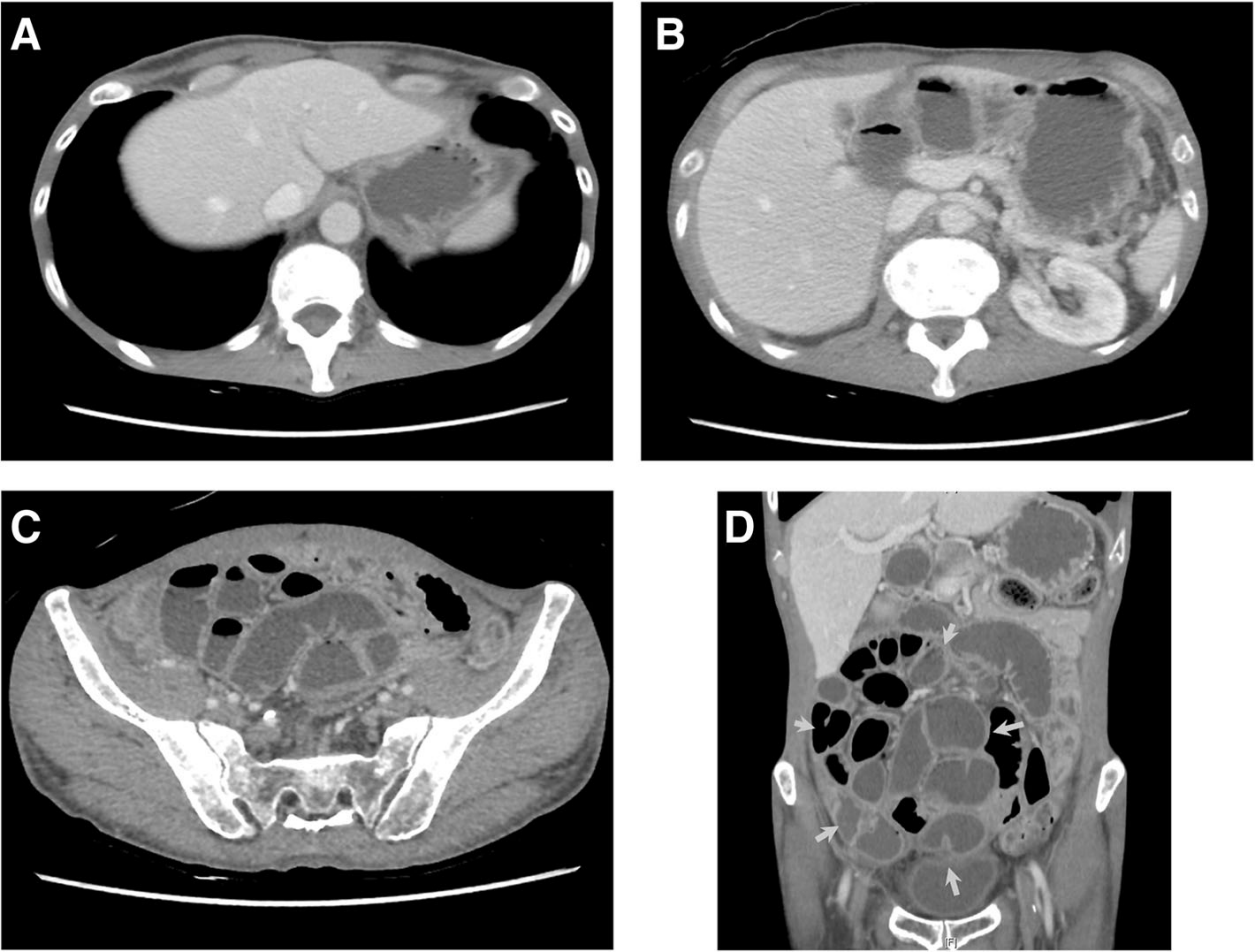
Computed tomography findings at the time of diagnosis of encapsulating peritoneal sclerosis. The patient had neither ascites nor pleural effusion (a, b). Computed tomography of the abdomen reveals dilated, thickened, adhered, and fluid-filled small bowel loops (c). A coronal reformatted computed tomography image shows that small bowel loops gathered to form a large mass as if they had a capsule (yellow arrows in d)
